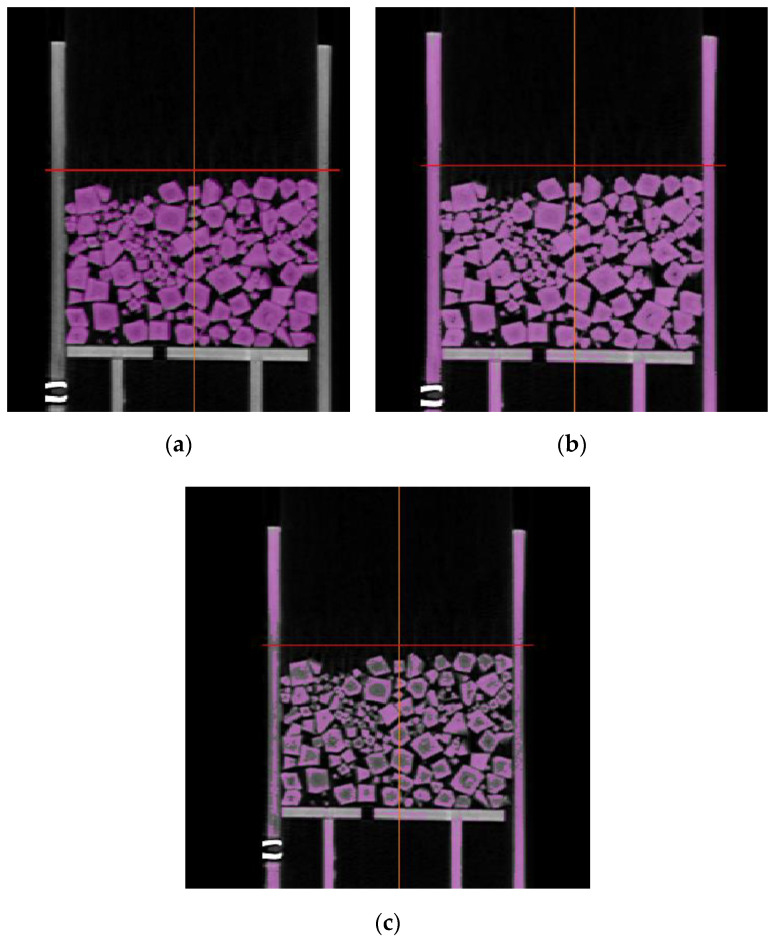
Results of the segmentation of the same CT image of O1 via different methods. (a) The SVM method. (b) The gray-scale morphology method. (c) The histogram segmentation method.
